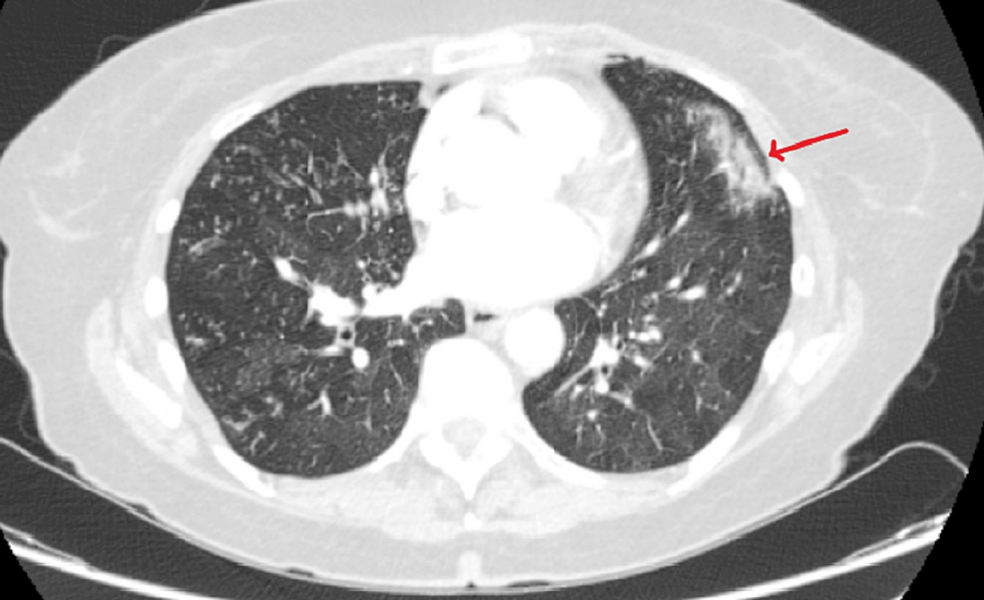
Axial CT pulmonary angiography slice showing non-specific changes The red arrow indicates the ground-glass opacity.
